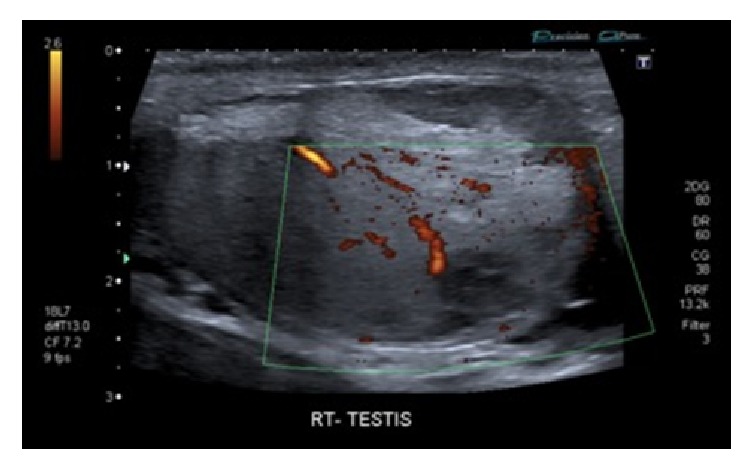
Fractured right testicle with Doppler flow to some areas on ultrasound scan.
